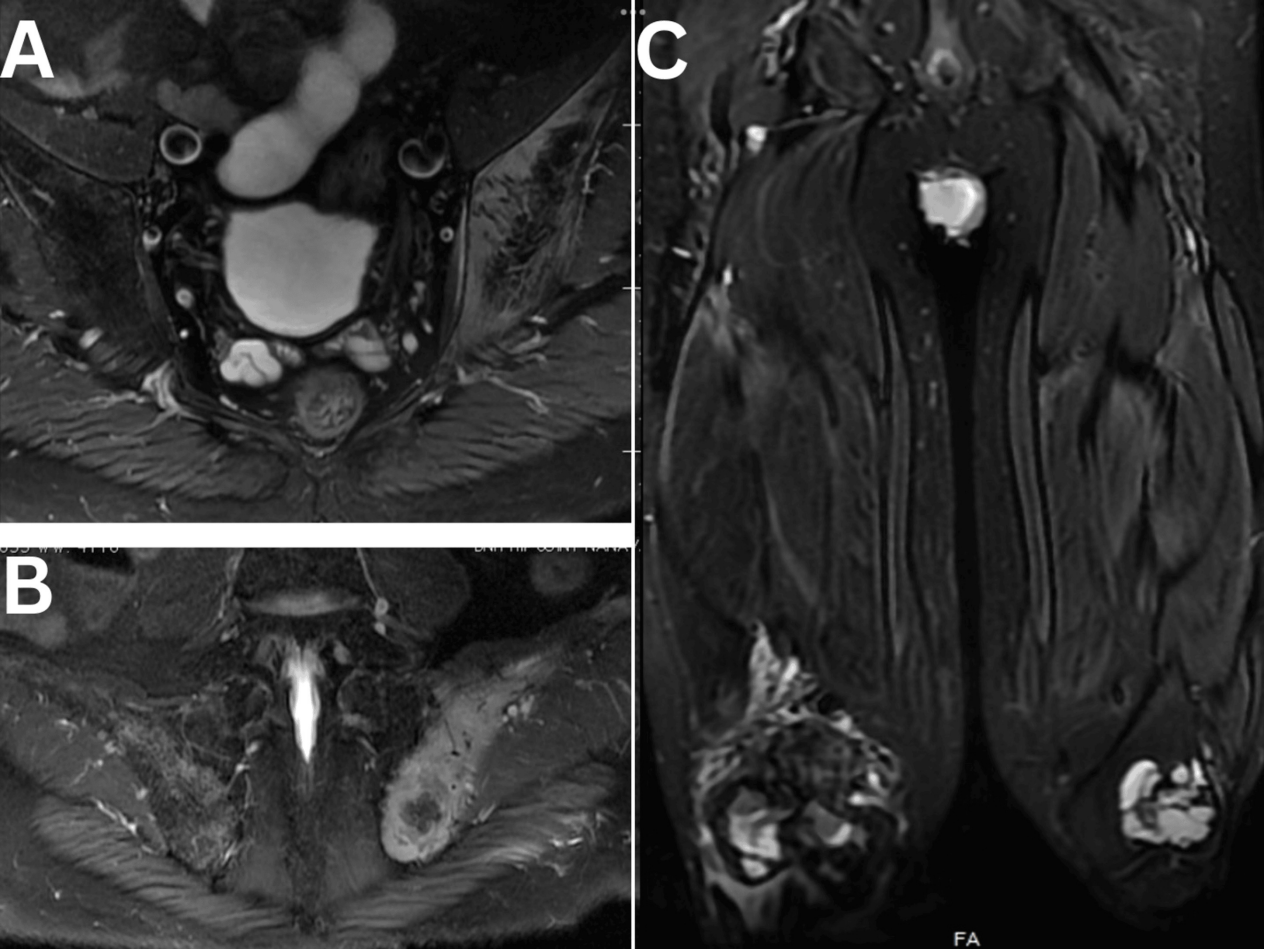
Plain MRI of the pelvis with bilateral hip joints: (A) axial fat-suppressed (FS) T2WI, (B) coronal FS T2WI. Images reveal a hyperintense lesion in the left iliac bone and sacroiliac (SI) joint, with involvement of the adjacent soft tissue and iliacus muscle. (C) Coronal FS T2WI of both thighs demonstrates multilobulated lesions in the bilateral femoral condyles with associated soft tissue involvement.
